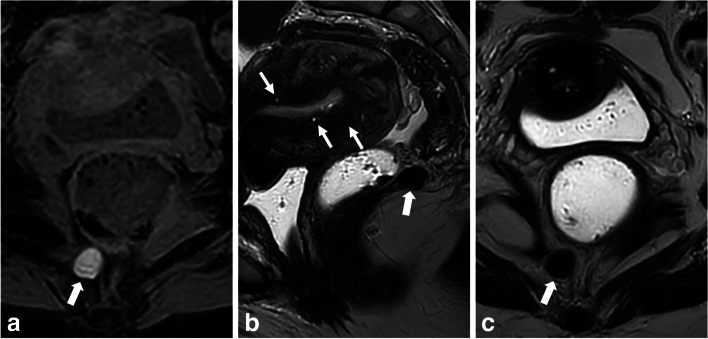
Retrorectal hamartoma (tailgut cyst) in a 46-year-old patient with gradually worsening dysmenorrhea and menometrorrhagia. a Axial fat-suppressed T1-W MR image shows a small-sized, well-defined and thin-walled T1-hyperintense cyst (thick arrow) isolated in the retrorectal space, along the right side of the anococcygeal raphe. b Sagittal and (c) axial T2-W MR images show a distinct T2-hypointense retrorectal cyst (thick arrows). Note the absence of posterior deep infiltrating endometriosis lesions but the presence of internal adenomyosis (thin arrows) consistent with the symptomatology
